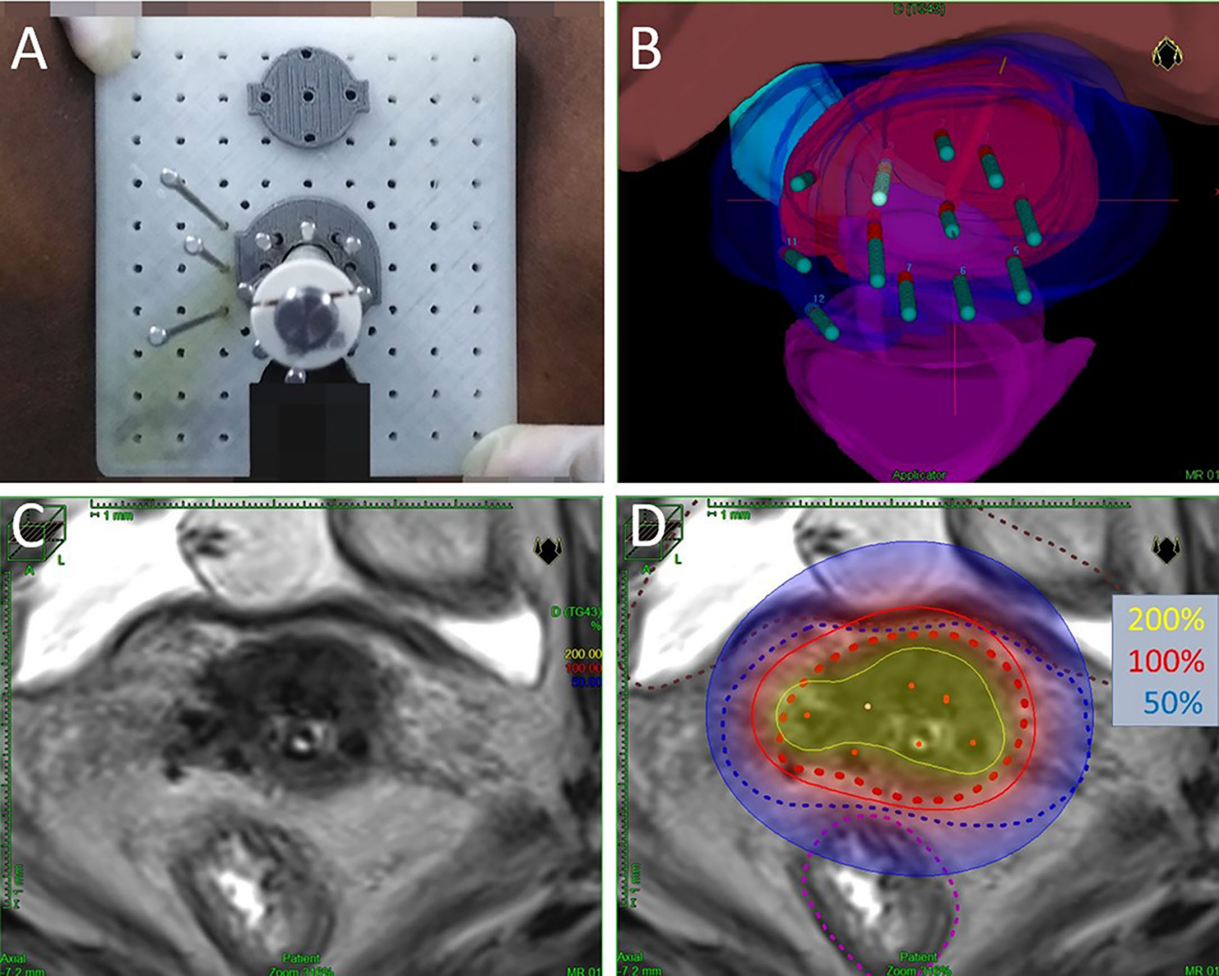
(A) Macroscopic view of self-made 3D-Printed applicator. (B) Three-dimensional view of the same implant planning data. The volumes represent HR-CTV (red), IR-CTV (blue), bladder (pink), rectum (purple), and sigmoid (cyan). (C) Axial view of T2-weighted magnetic resonance images (with implant *in situ*). (D) Axial view of brachytherapy dose distribution. Dotted red line is HR-CTV, dotted blue line is IR-CTV, dotted brown line represents the bladder, and dotted purple line represents the rectum. The isodose lines color code conventions are: solid yellow line = 200%; solid red line = 100%; solid blue line = 50% per treatment fraction.
